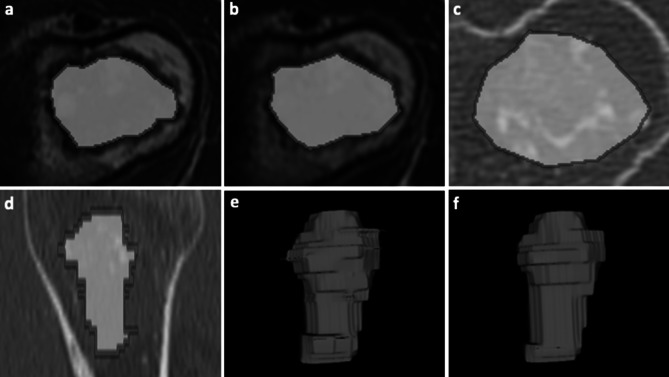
Contour-focused and margin shrinkage segmentation of an atypical cartilaginous tumor of the humerus in a 45-year-old woman. a–c 2D contour-focused segmentation was performed on axial T1-weighted MRI a, T2-weighted MRI b, and bone-window CT c on the slice showing the largest tumor extension. d 3D contour-focused segmentation was performed slice by slice in the axial plane to include the whole tumor volume, as shown in the sagittal CT image. Contour-focused segmentation provided the ROI including both green and red areas. Margin shrinkage segmentation provided the ROI including only the green area by computing a marginal erosion, which is shown in red. e–f Segmented tumor volumes obtained with 3D contour-focused e and margin shrinkage f segmentation are shown, where the latter has smoother margins as a result of marginal erosion
